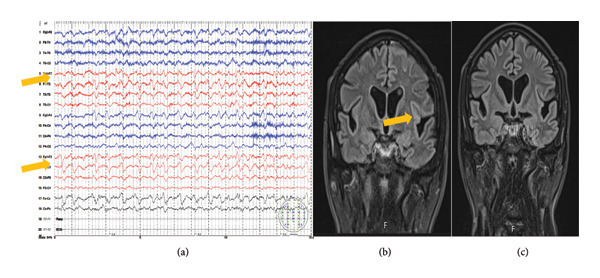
(a) Standard EEG showed ictal rhythm under the left FCT region, (b) brain MRI confirmed a T2w/FLAIR hyperintensive lesion in the left opercular‐insular region, and (c) follow‐up MRI with complete regression of previously verified lesion.
